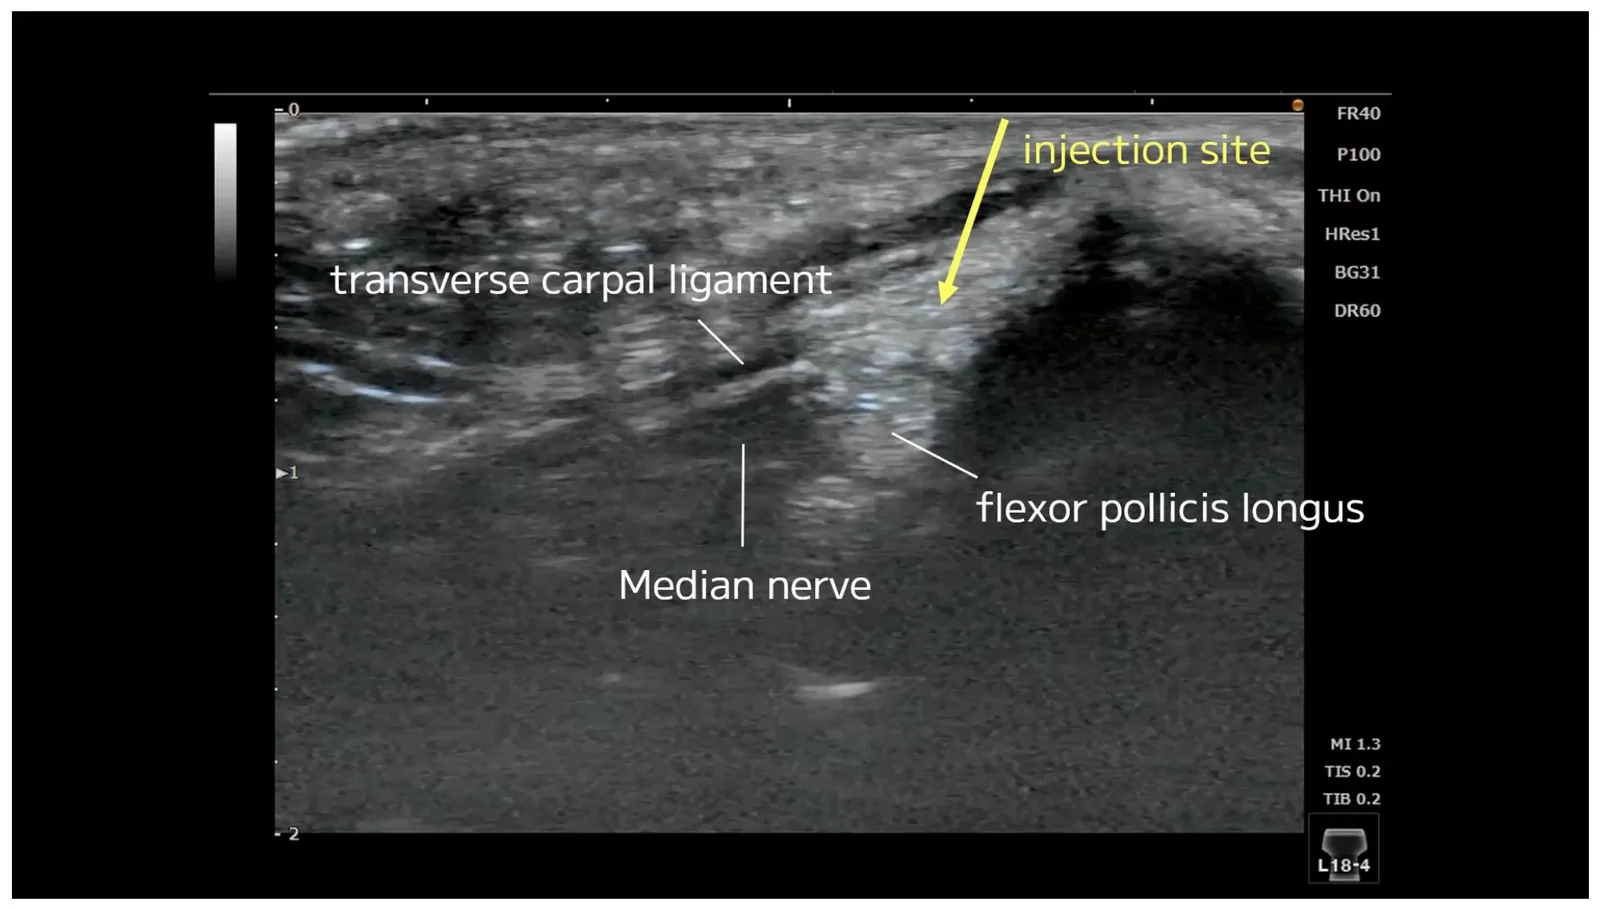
Point 14—median nerve/flexor pollicis longus (carpal tunnel): ultrasound-guided fascia hydrorelease; yellow arrow indicates the injection site.
